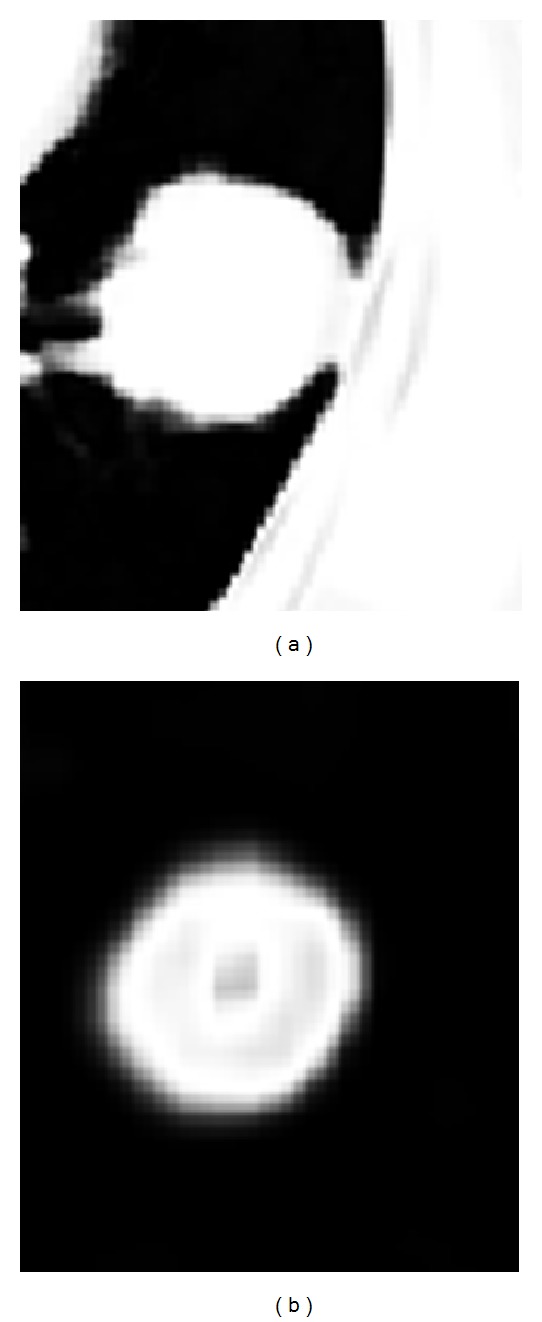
Membership degrees to tumor class of the selected region in Figure 2 obtained with fuzzy *C*-means clustering; (a) only CT intensity feature is used; (b) only SUV feature is used.
